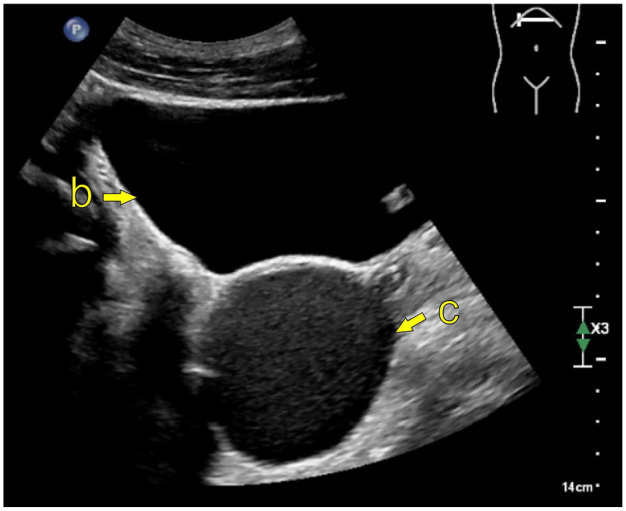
Transabdominal ultrasound; EPIQ Elite (Philips Medical System); curved transducer C5-1. (b) Bladder, (c) Cystic enlargement of the left seminal vesicle.
